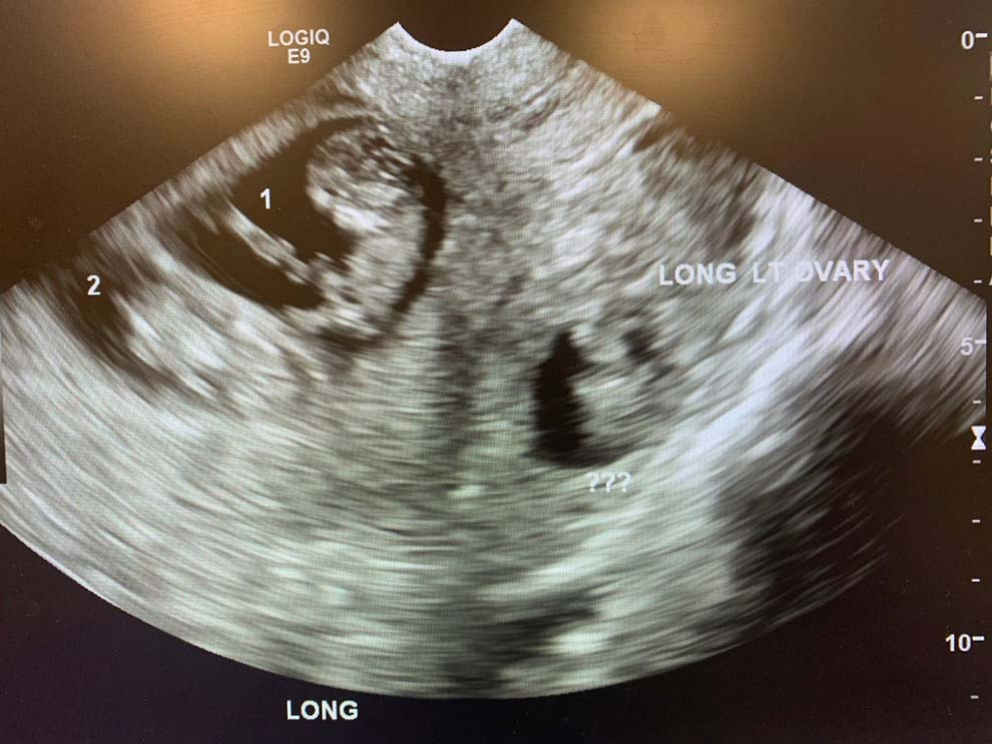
Transvaginal ultrasound view of heterotopic pregnancy.
